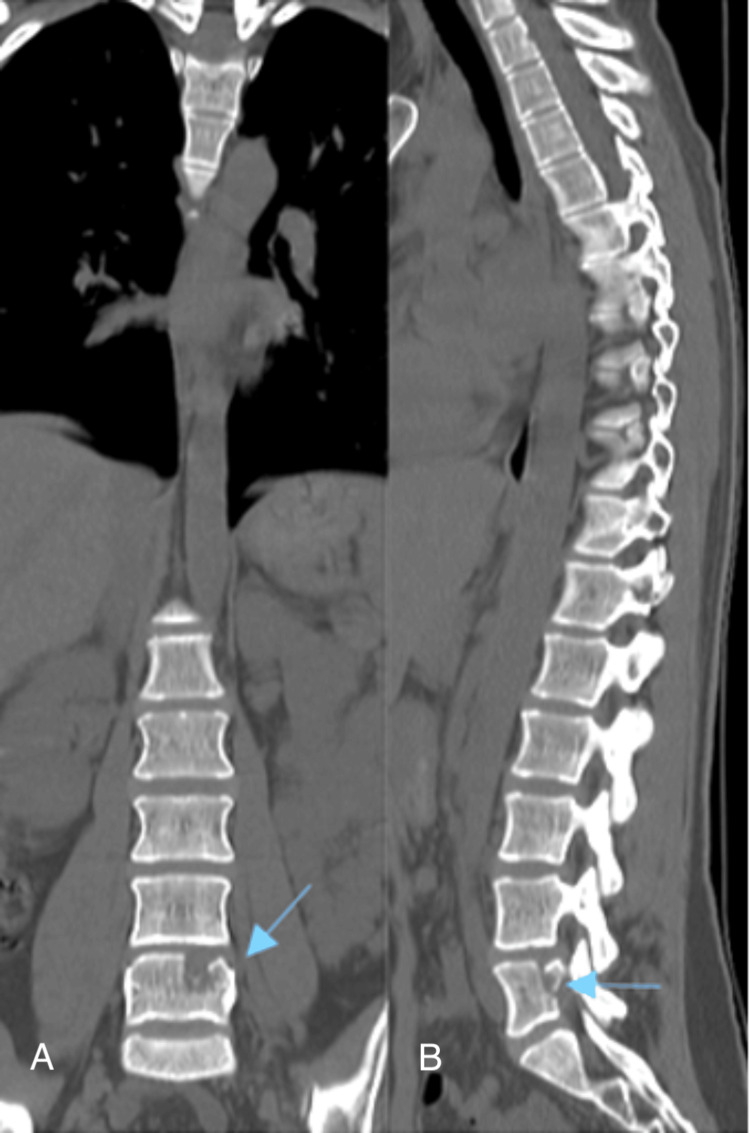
Lumbar CT scan A: coronal; B: sagittal views. The blue arrows indicate bone depression and deformation at the L4/L5 level.
